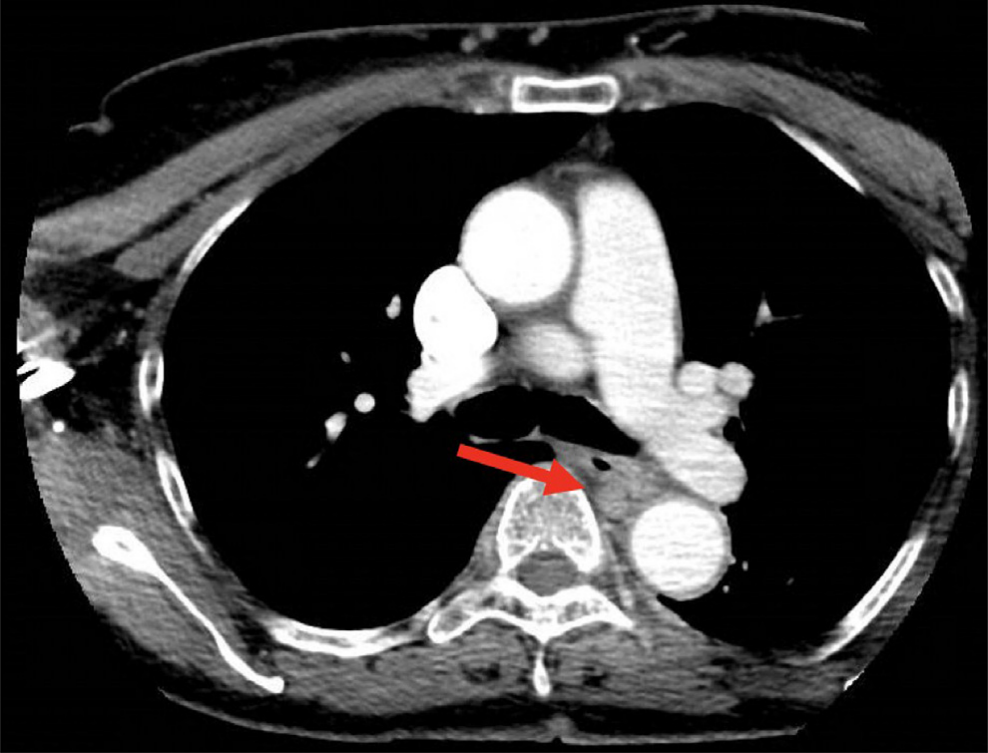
CT neck with contrast demonstrates a mass compressing the esophagus at the level of the carina.
